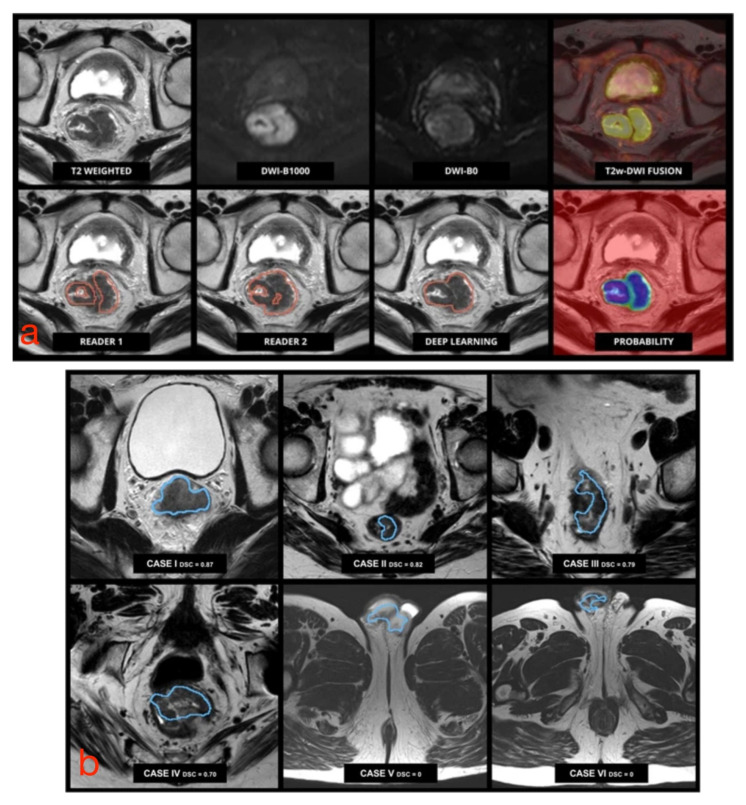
(a) Pretreatment multiparametric rectal MRI examination in a male patient with RC. Upper row (from left to right): axial MR images from T2-weighted images, DWI b1000, DWI b0, and fusion imaging between T2-weighted and DWI b1000 images. Lower row (from left to right): tumor segmentation performed by an experienced reader used for training, an independent reader, the algorithm output, and the corresponding probability map generated by the algorithm. (b) Performance of CNN-based segmentation. The algorithm correctly identified and segmented the tumor in cases I to IV (small field of view), but it failed with a larger field of view images (V and VI), where parts of the cavernous bodies of the penis were mistakenly included in the segmentation. Adapted from [46].
